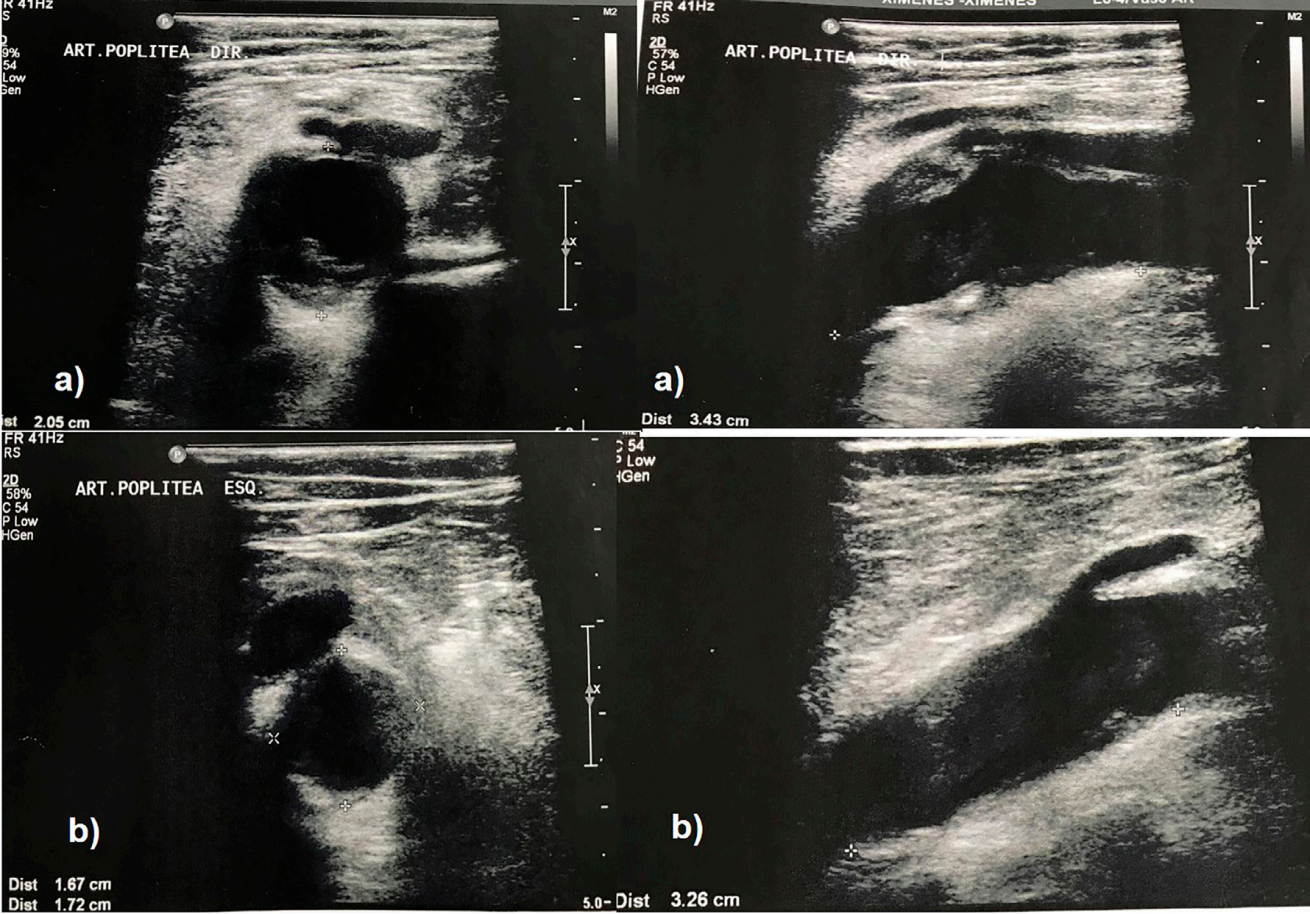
Aneurysmal dilatation of the right (a) and left (b) popliteal arteries, with mural thrombus visible on ultrasound.
